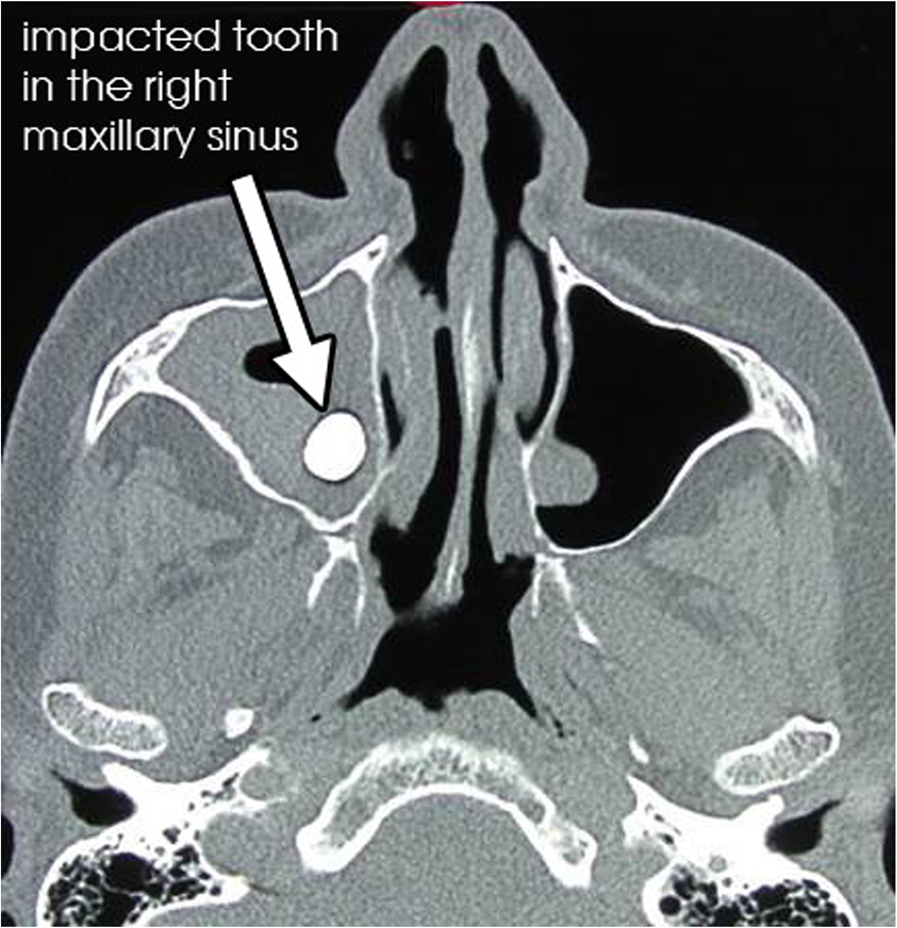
Axial computed tomography image showing a homogenous soft tissue mass occupying the entire right maxillary sinus with an impacted tooth.
